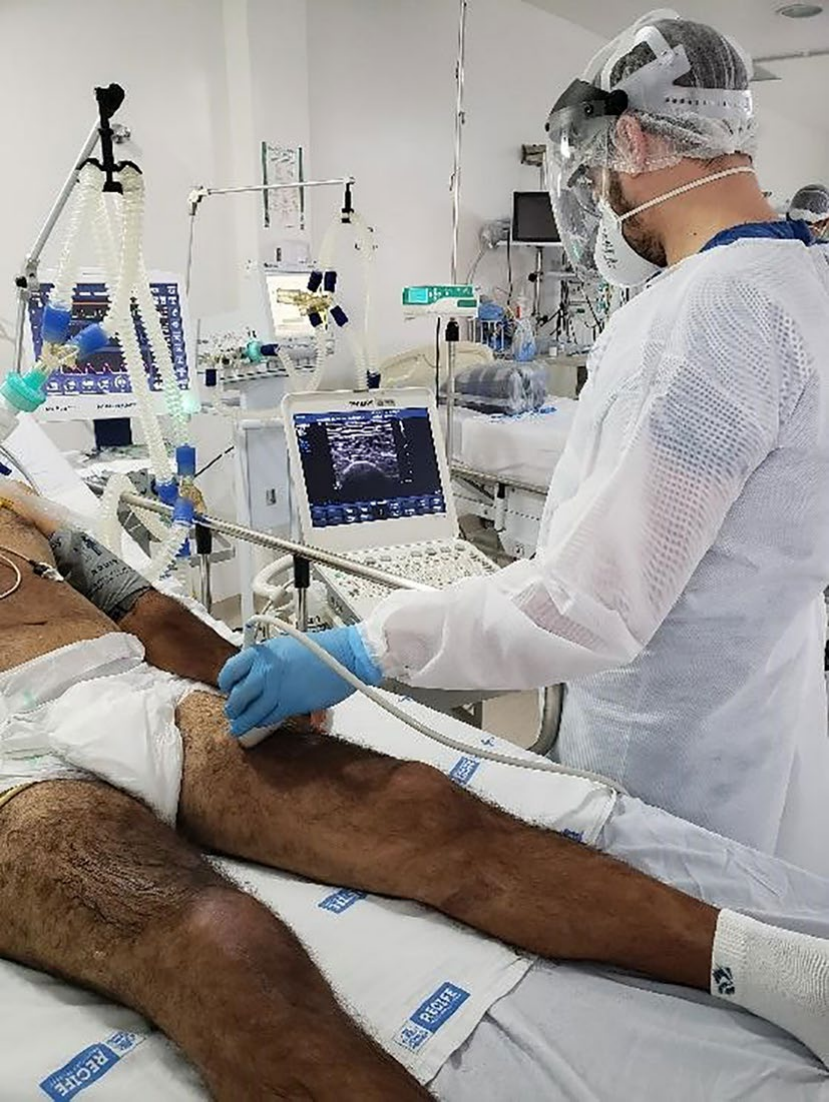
Ultrasound evaluation in the intensive care unit.
